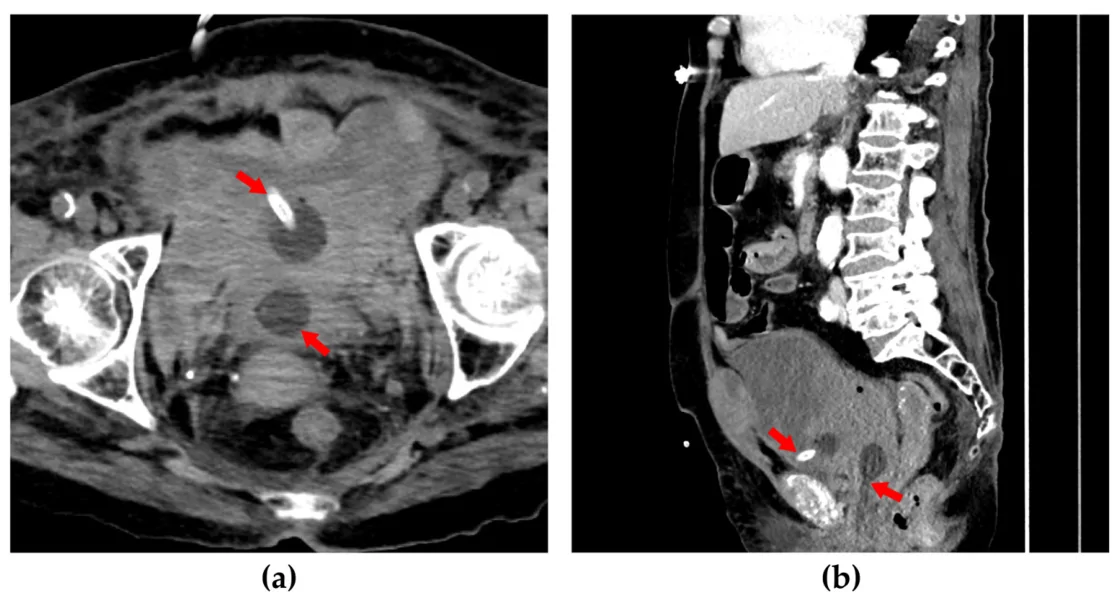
CT urography in (a) axial and (b) sagittal views. The upper red arrow indicates the cystostomy tube, and the lower red arrow points to the urinary catheter.
